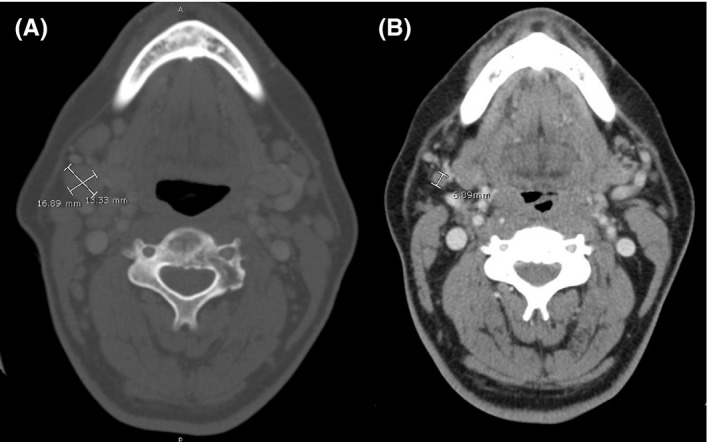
CT scan of the neck shows an enlarged right submandibular lymph node before (A) and after (B) R‐CHOP. The lymph node is significantly smaller in size after ten weeks (three cycles) of chemotherapy
